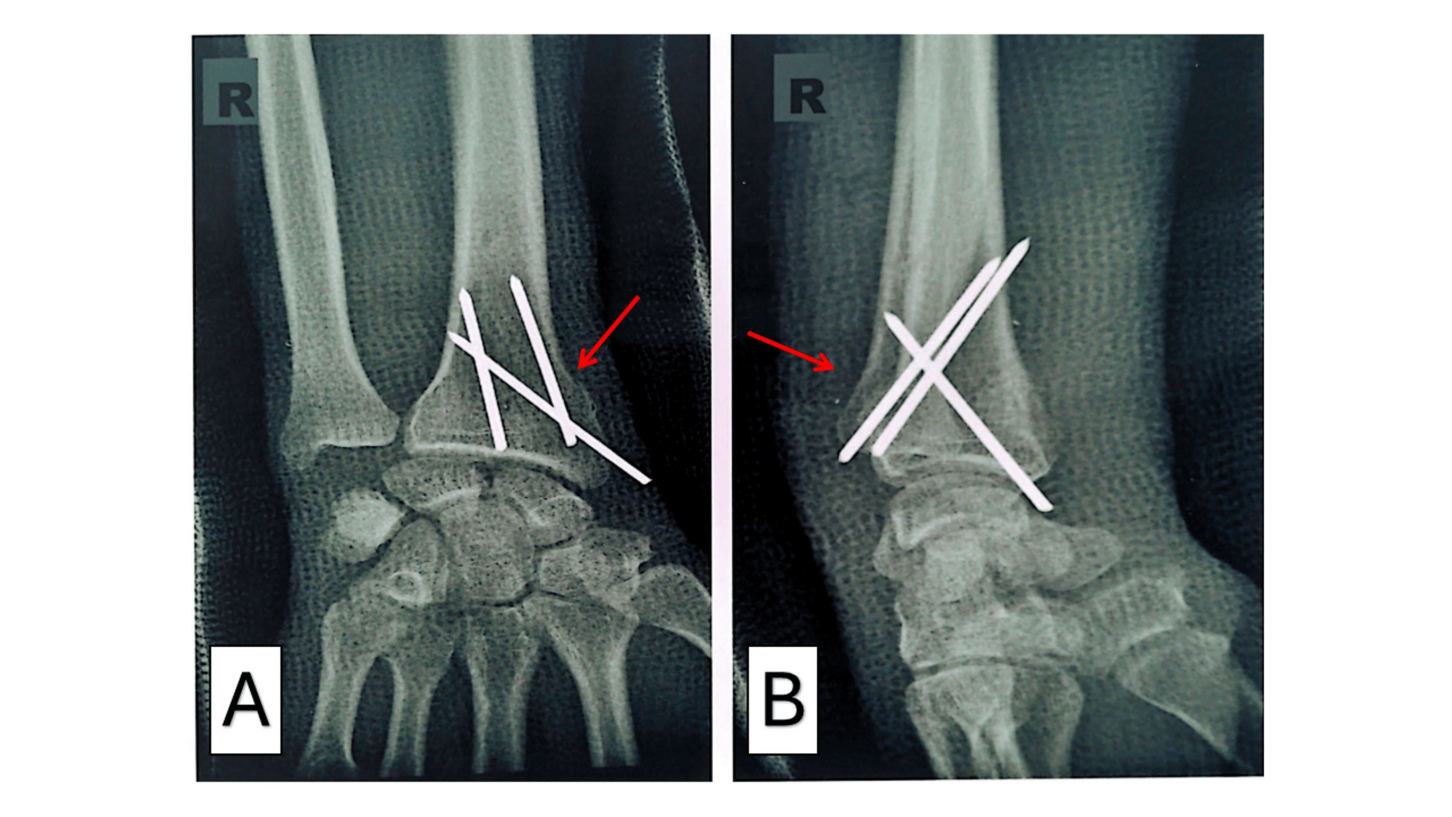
Postoperative anteroposterior (A) and lateral (B) radiographs showing reduction and osteosynthesis of the distal radius fracture using three pins placed with a freehand technique A (anteroposterior view) and B (lateral view) show postoperative plain radiographs of the wrist following closed reduction and percutaneous pinning of a distal radius compression fracture. The original injury was an extra-articular compaction/impaction fracture with dorsal cortical buckling and mild dorsal angulation of the distal fragment (Colles’ type pattern). Post-reduction alignment is restored with anatomical radial height, inclination, and near-neutral tilt. Fracture stability is achieved using three smooth percutaneous Kirschner wires (K-wires): Two parallel radial styloid pins were inserted from the dorsal-ulnar aspect of the styloid, engaging the opposite intact cortex (clearly visible in Figure A). One dorsoulnar pin crosses the fracture site obliquely, providing three-point fixation and buttressing the dorsal comminution (best seen in Figure B). The pins maintain fracture reduction without loss of position. No intra-articular penetration is observed. The configuration effectively neutralizes compressive and bending forces, ensuring stable fixation during healing.
